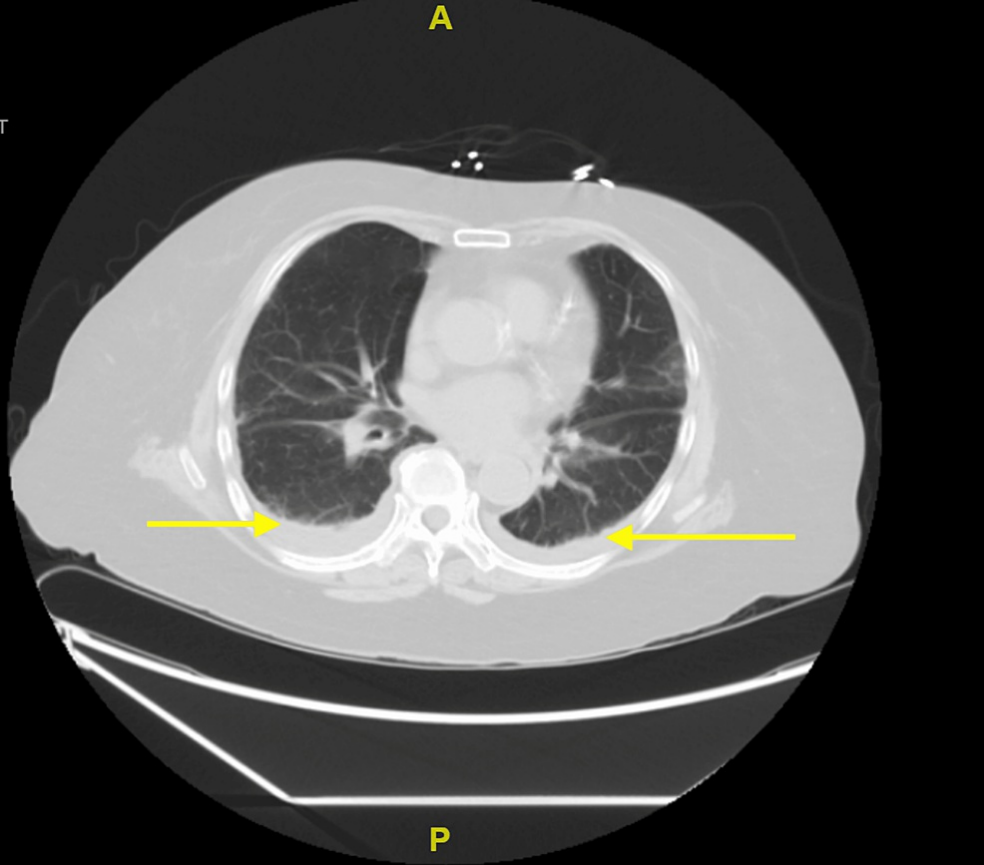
CT of the chest demonstrated small bilateral pleural effusions and possible pneumonitis, suggesting MTX toxicity. CT, computed tomography; MTX, methotrexate
